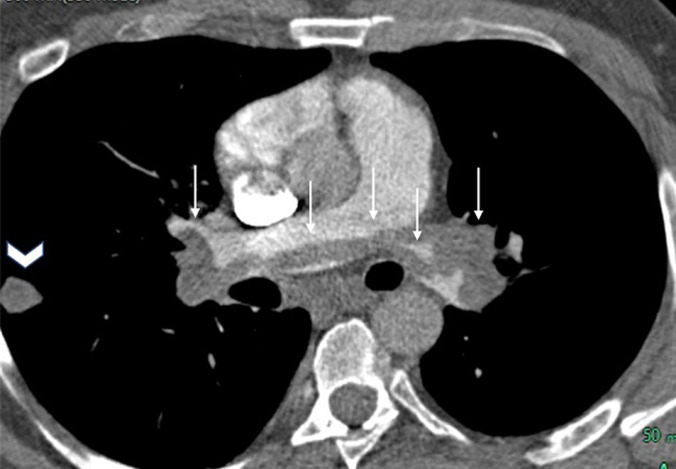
CT pulmonary angiogram mediastinal window axial coronal oblique view demonstrating saddle embolus involving distal main pulmonary artery and extending into both left and right pulmonary arteries ( white arrows) and a well-defined right lung lateral subpleural opacity (white arrow head)
